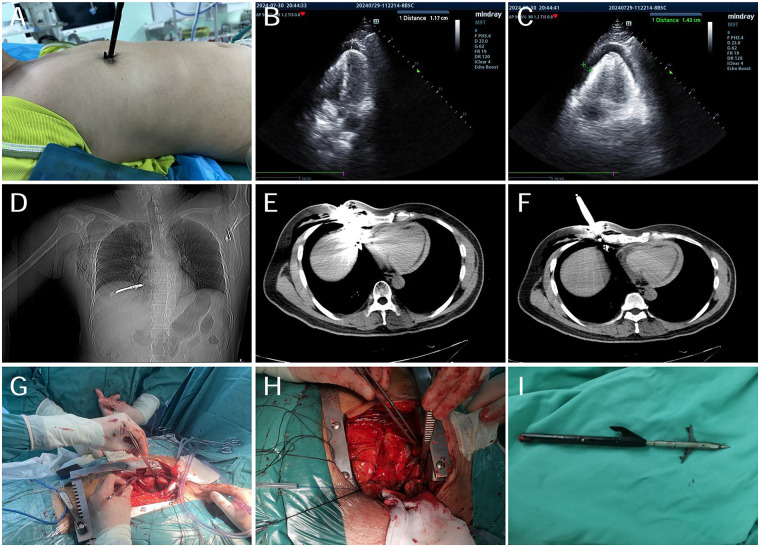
(A) physical examination revealed a cylindrical foreign body approximately 8 cm in length obliquely inserted into the right fourth intercostal space of the chest wall, close to the nipple area, that moved with respiration. (B,C) Echocardiography showed a fluid-filled dark area in the pericardial cavity, with a distance of 14.3 mm on the right ventricular side and 11.7 mm at the apex, suggesting cardiac tamponade. (D–F) Enhanced chest CT scan showed that the foreign body in the right thoracic cavity penetrated the chest wall, the middle lobe of the right lung tissue, and the pericardium, and the proximal end touched the right atrial wall. (G–I) During the procedure, the foreign body was identified as a cylindrical shark dart. The object had penetrated the chest wall, traversed the middle lobe of the right lung and the pericardium, and ultimately reached the right atrium. The shark dart was successfully removed by thoracotomy.
